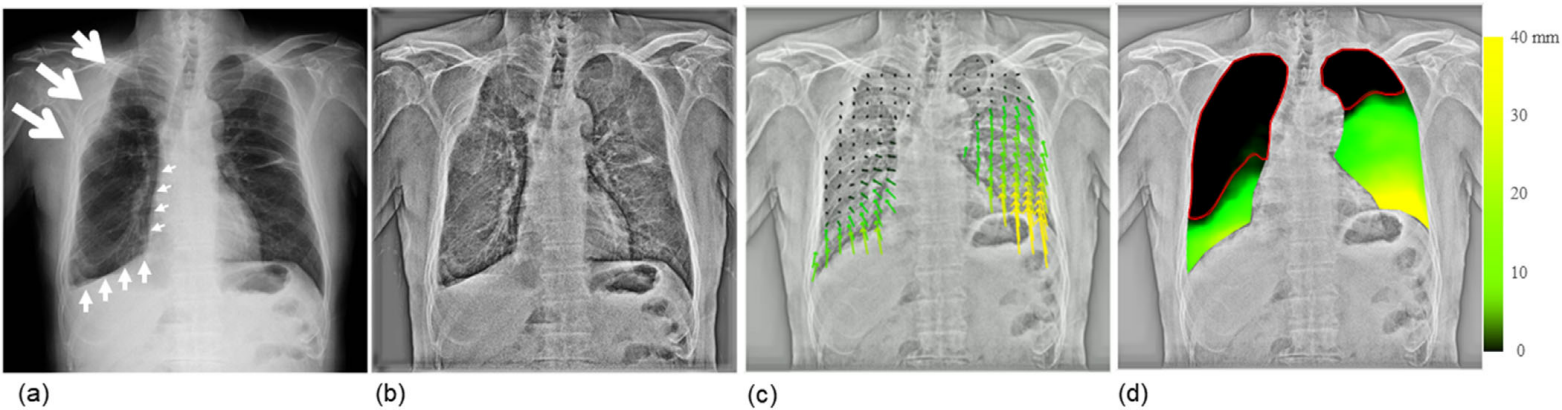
An example of a true‐positive case correctly evaluated as showing pleural adhesion by dynamic chest radiography (DCR)‐based quantitative motion analysis. Frame of the original DCR image (a), bone‐suppressed DCR with enhanced high spatial frequency (b), vector‐map images (c), and displacement‐map image (d) of a 69‐year‐old man with right lung cancer (grade 4 pleural adhesion over the right lung). The patient showed poor motion in 69.9% and 27.3% of the right and left lung areas, respectively, with a predicted VC of 73.9%, predicted FEV1 of 84.9%, FEV1/FVC ratio of 84.2, and predicted DLco of 55.3%. White arrows indicate areas with pleural invasion or adhesions; large, middle, and small arrows indicate invasion and strong and moderate adhesions, respectively. DLco, diffusing capacity of the lung for carbon monoxide; FEV1, forced expiratory volume in 1 s; FEV1/FVC, forced expiratory volume in 1 s/forced vital capacity; VC, vital capacity.
